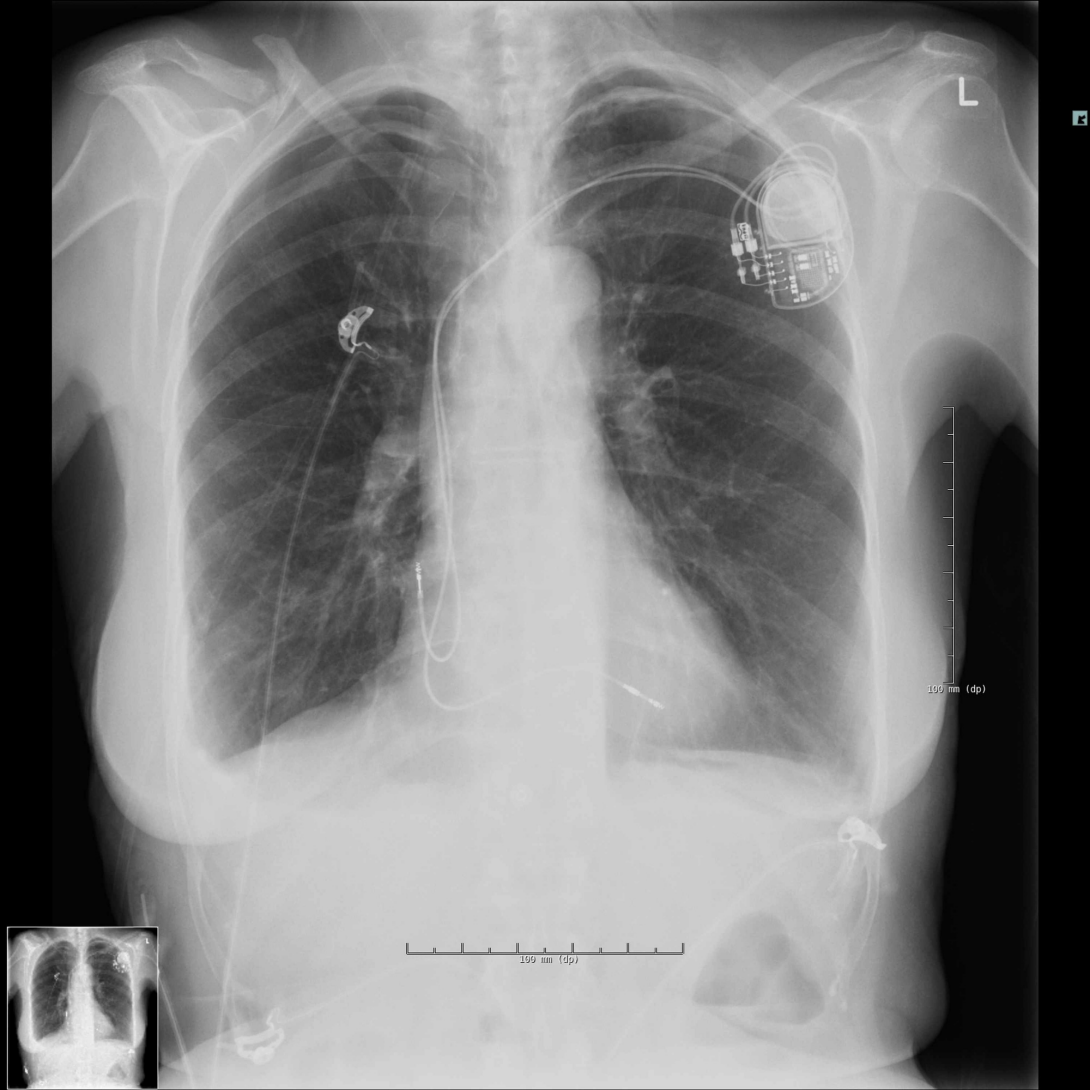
Chest X-ray the day after implantation of the pacemaker (day 2).
